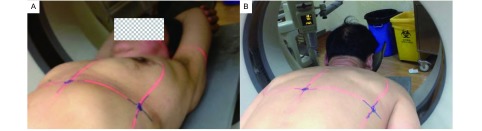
患者行胸部CT扫描姿势。A：仰卧位；B：俯卧位。 Position of patients underwent CT scan. A: Supine position; B: Prone position. CT: computed tomography.
